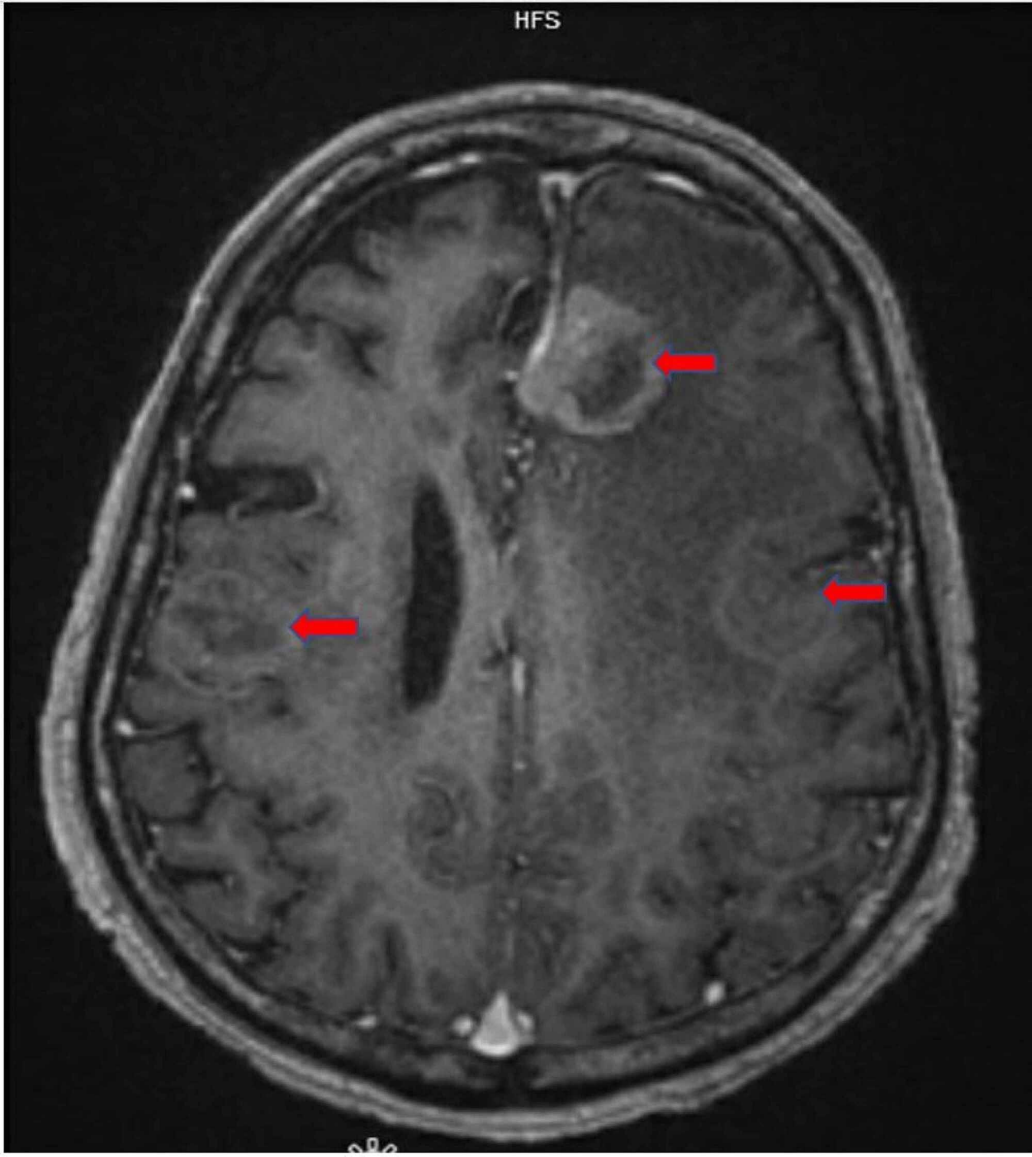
MRI brain with contrast, axial section. Red arrow showing multiple lesions with surrounding edema.
